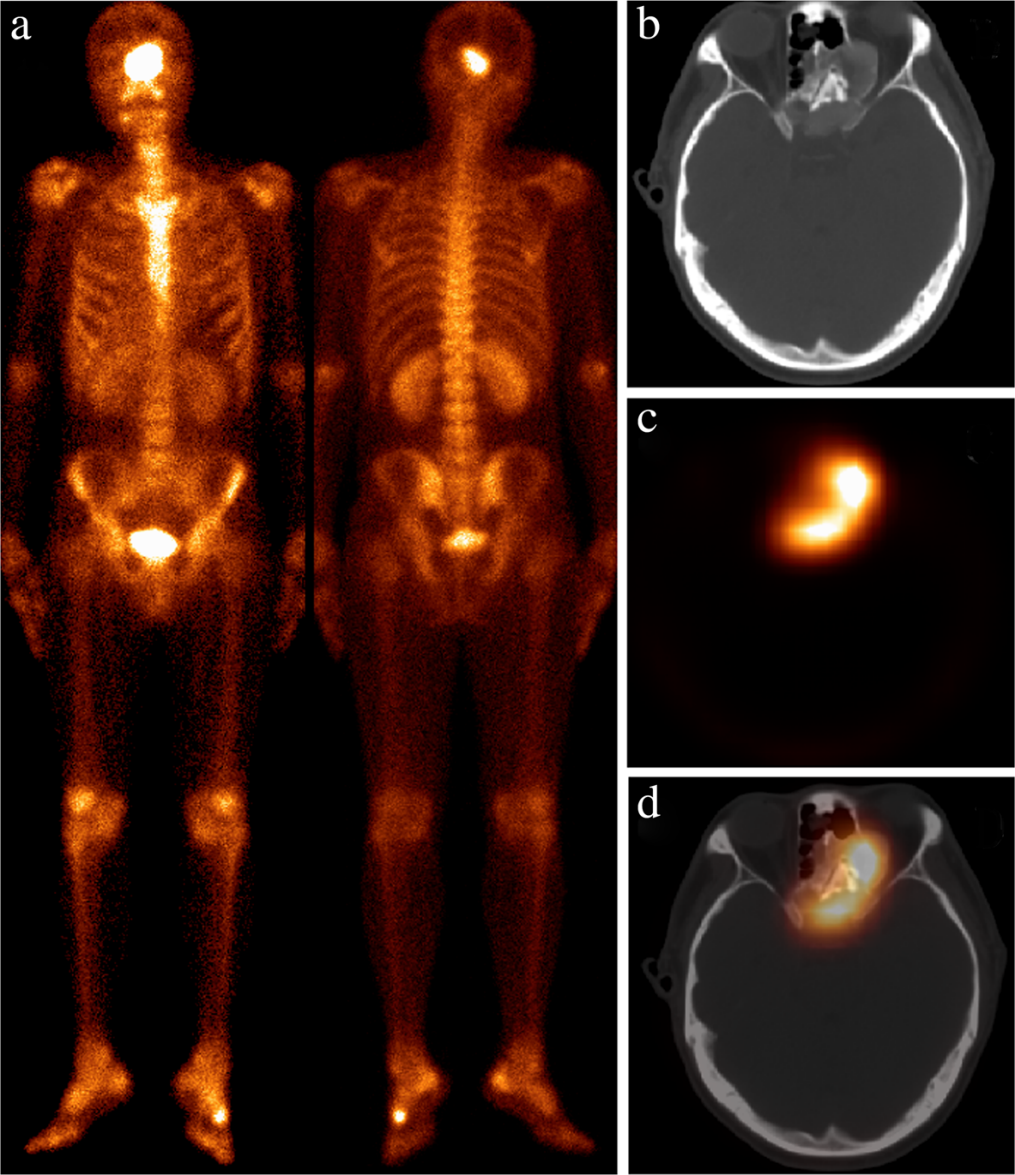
Patient 5 presented with HCC. The WBS (a) demonstrated markedly increasing ^99m^Tc-MDP uptake in the base of skull. Axial CT (b), SPECT (c), and hybrid SPECT/spiral CT imaging (d) showed an expansile lesion, which presented with GGO and ill-defined borders in the conjoint sphenoid and ethmoid. The clinician decided immediately to perform a biopsy, because the anatomic site of the lesion was easily accessible by endoscopic sinus. Pathologic analysis confirmed the diagnosis of FD
